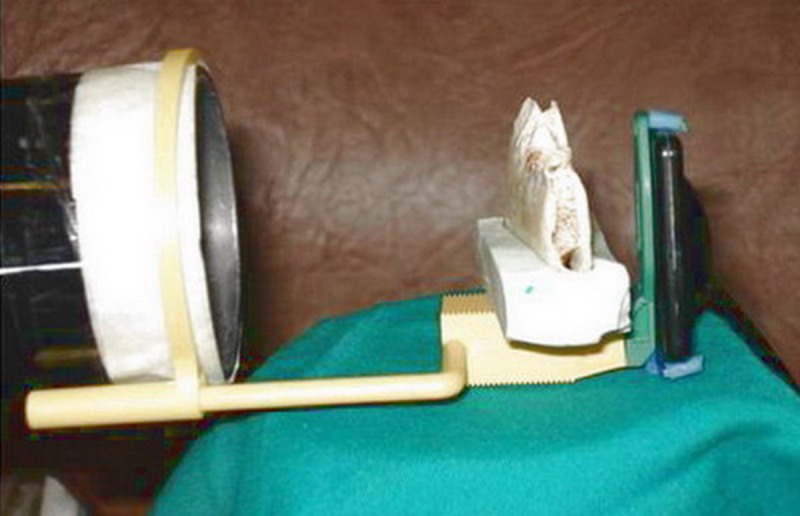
The focus-receptor distance was set at 20 cm and radiographs of the teeth were taken in an orthoradial direction. All images were exposed with a Minray radiographic machine (SOREDEX, Tusula, Finland) operated at 70kVp, 8 mA and exposure times of 0.03 and 0.12 seconds for PSP and CCD sensors, respectively, based on a pilot study for optimal image quality. Two digital sensors were used: Dr. Suni CCD detector (Suni Medical Imaging, San Joe, USA) size 2, offering 2 megapixels and pixel size of 22 μm with a resolution of 23 Lp/mm; and PSP detector (Digora^®^Optime; SOREDEX, Tusula, Finland) size 2 and scanner 40 μm (pixel), offering 12.5 Lp/mm spatial resolution. The exposed phosphor plates were immediately scanned.
